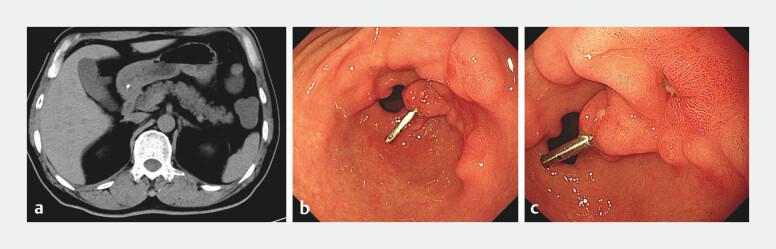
Computed tomography (CT) and gastroscopy images at the 3-month follow-up.
